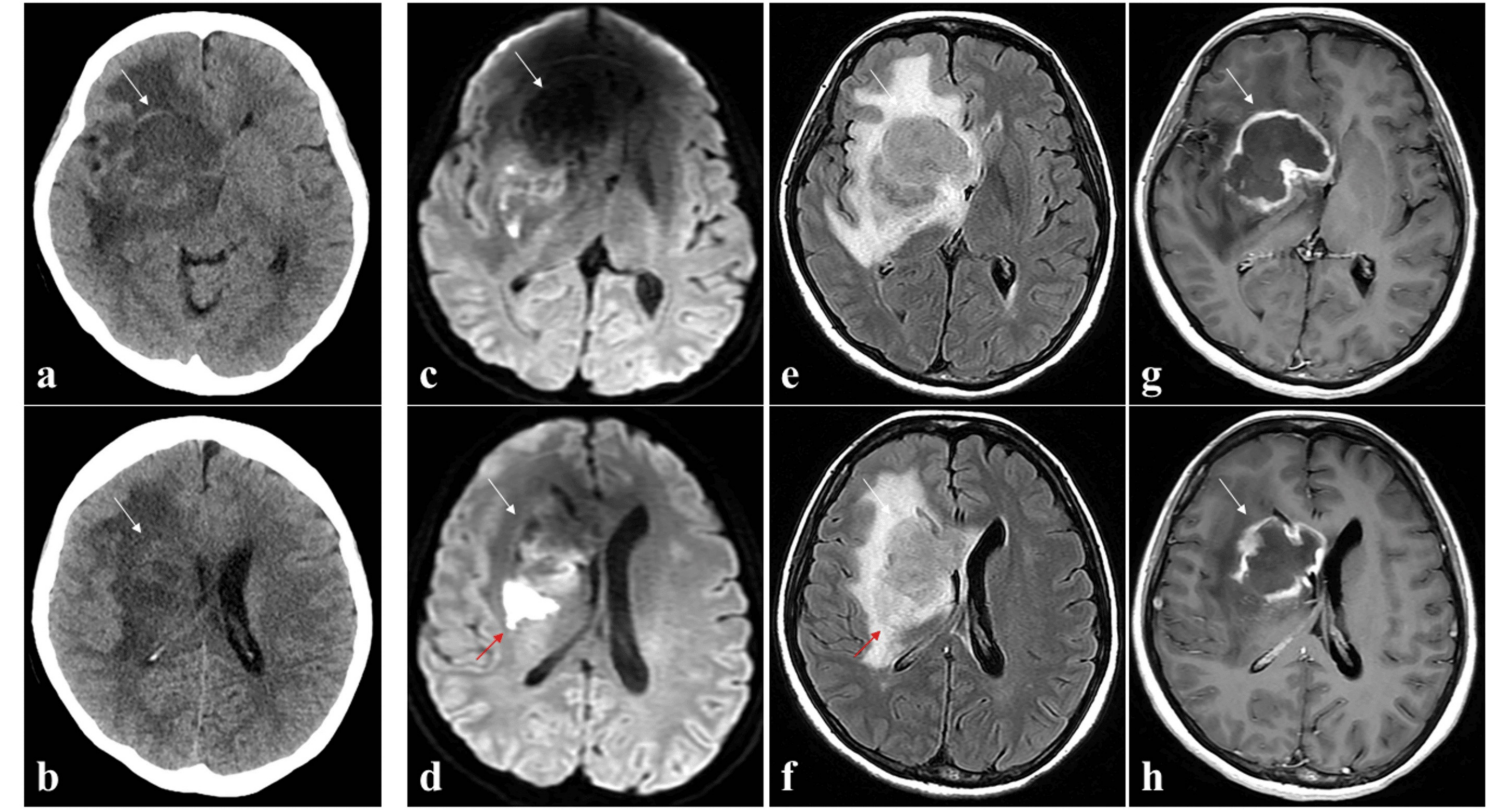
Computed tomography (CT) and magnetic resonance imaging (MRI) on admission. CT images (a, b) without contrast enhancement show an abnormal mass (white arrow) in the right frontal lobe and basal ganglia. Preoperative axial diffusion-weighted images (DWI) (c, d), fluid-attenuated inversion recovery images (FLAIR) (e, f), and gadolinium (Gd)-enhanced T1-weighted images (g, h) show an intracranial mass with ring enhancement (white arrow). In addition, the right corona radiata exhibited areas of restricted diffusion on DWI, which were interpreted as cerebral infarction (red arrow: d, f).
